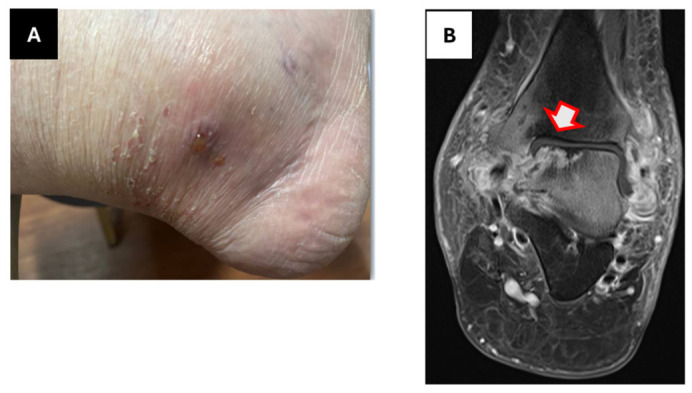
Osteoarticular coccidioidomycosis. (A). Swollen left ankle with punctate area from which purulent material had drained. (B). MRI of left ankle showing T1 hypointensity, marrow edema, and enhancement of the talus and adjacent distal tibia, and osseous destruction at the medial talar dome and posterior distal tibia (arrow).
